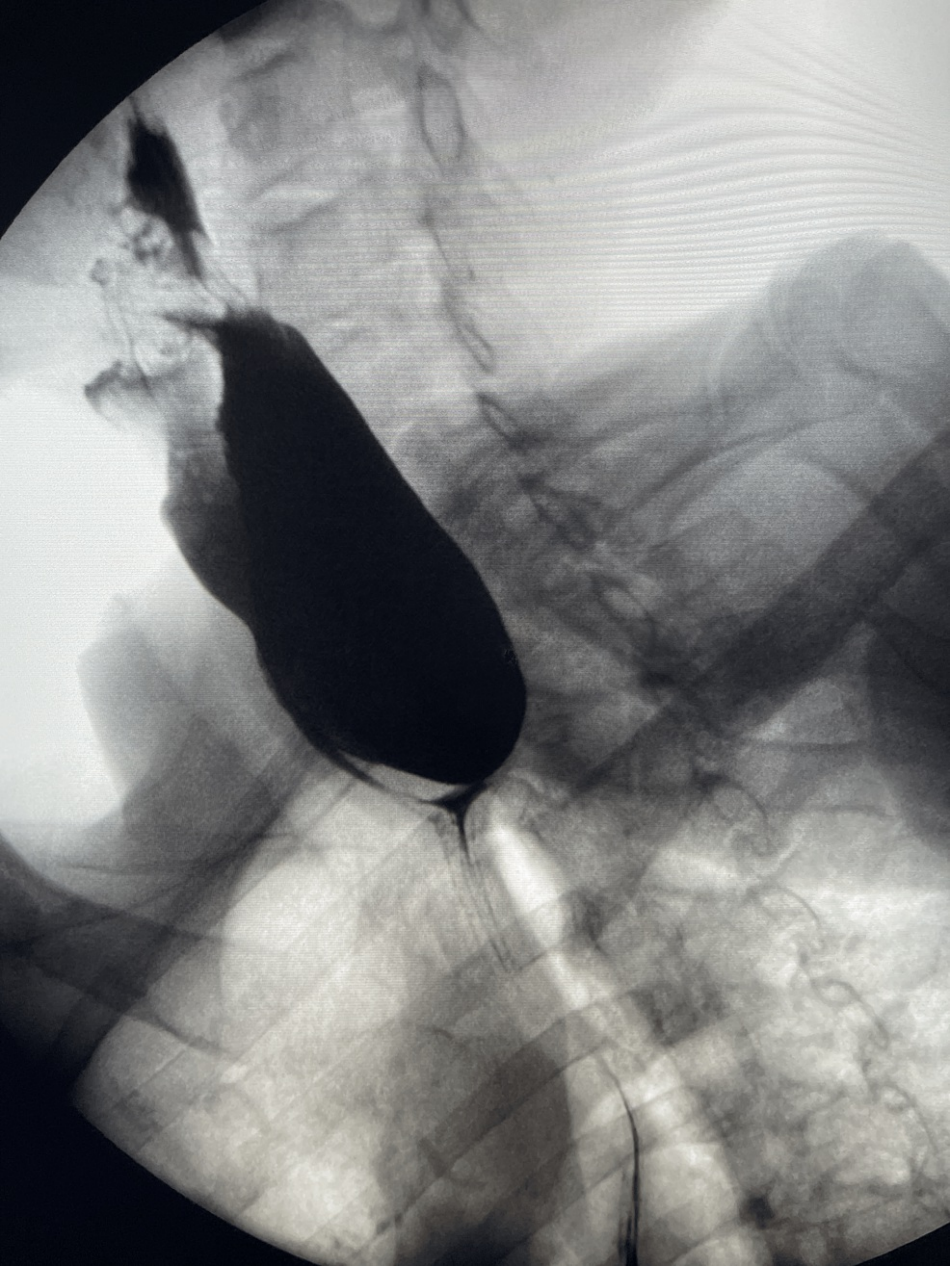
Preoperative barium swallow radiograph exhibits significant retention of barium
